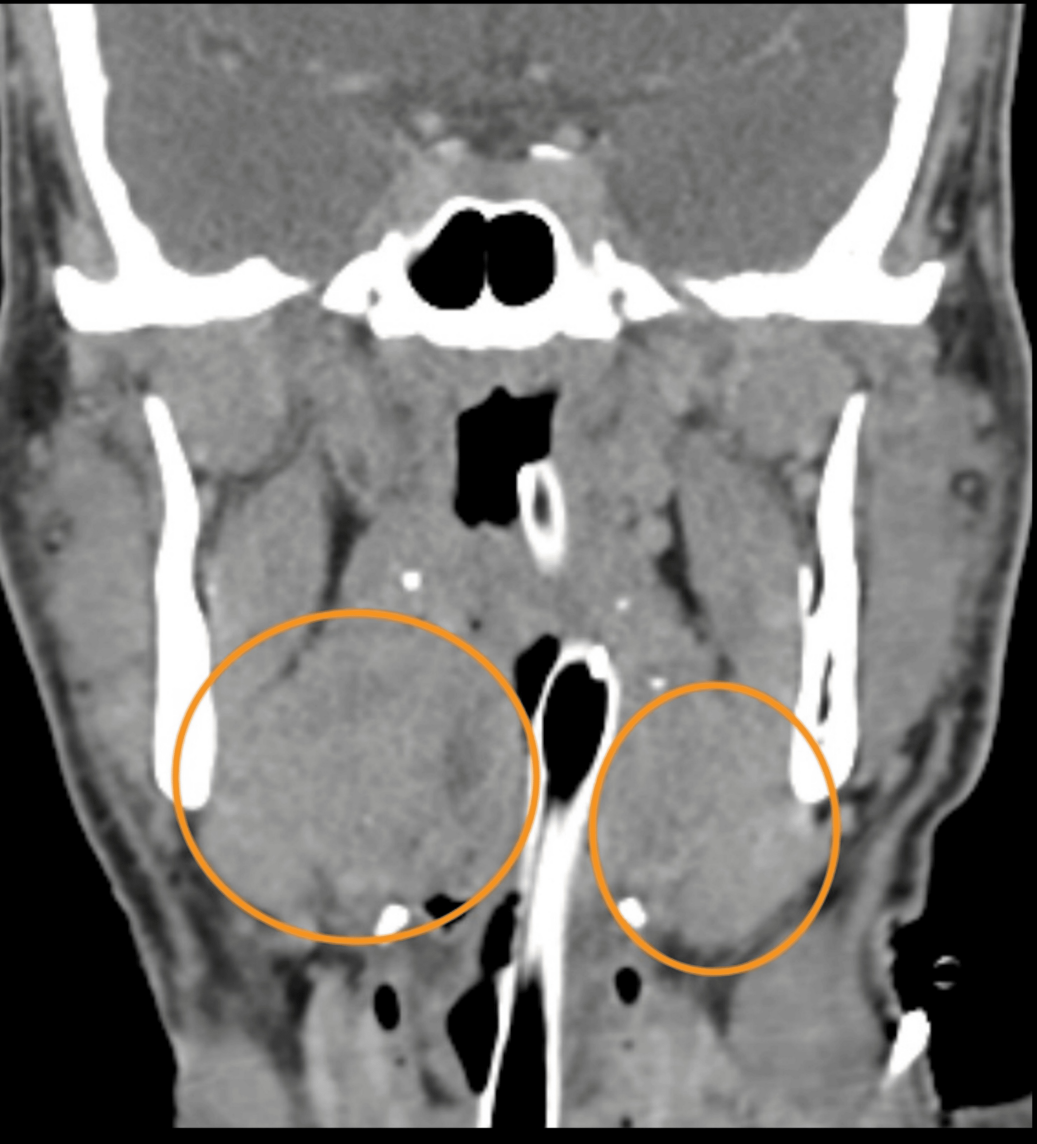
Neck CT scan showed an enlarged palatine tonsil (orange circle), without local infectious complications The tonsils are marked with orange circles. In the left circle: enlarged tonsil with no local complications. In the right circle: slight enlargement of the tonsil, with no local complications.
